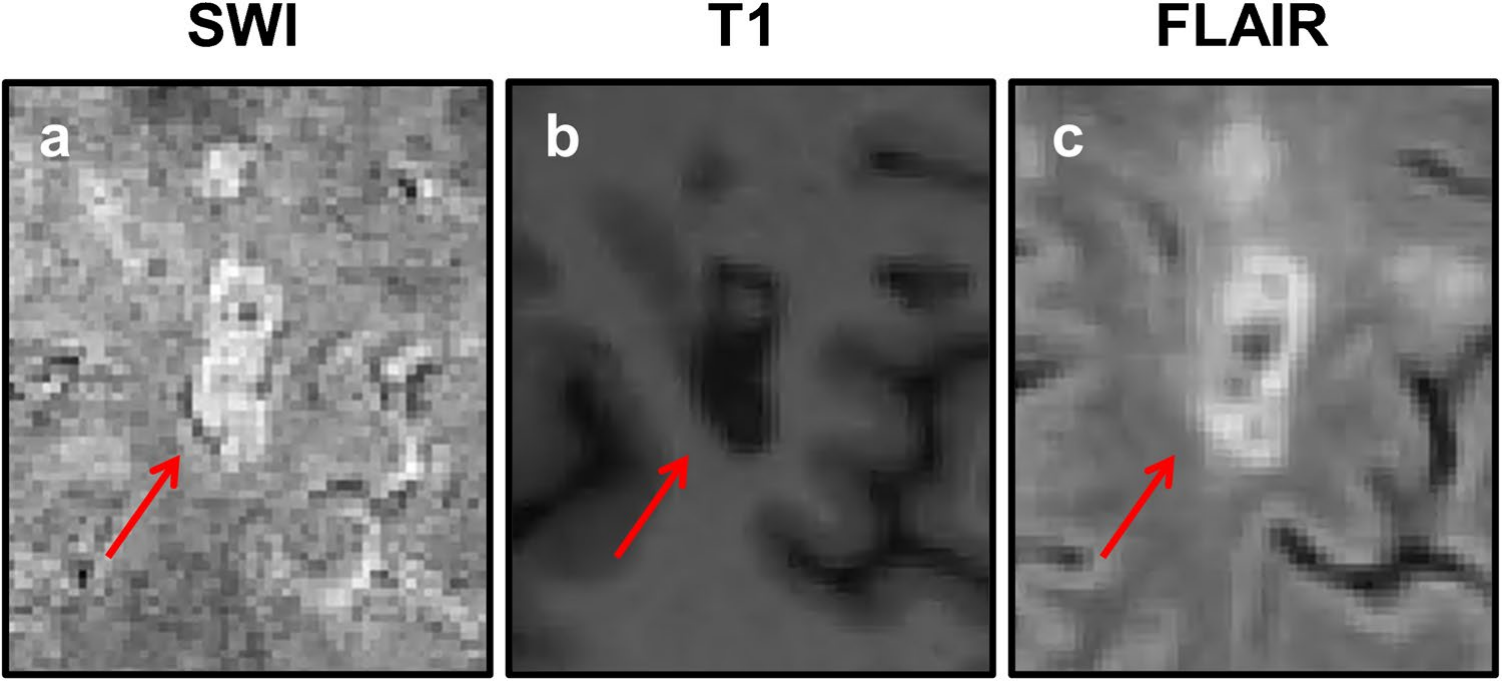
T1 and FLAIR appearance of an SWI rim lesion. All rim lesions observed in our MS cohort, including this white matter lesion (indicated by red arrow) exhibited T1 hypointensity. The lesion shown above is one of ten rim lesions that on FLAIR also appeared hyperintense with a core isointense or in this case, hypointense, relative to extralesional white matter
